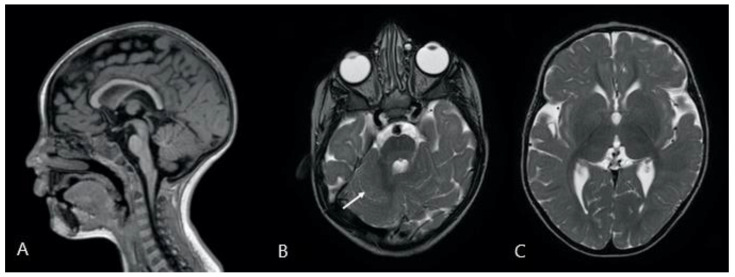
Description: (A): 3D-T1-weighted midline sagittal image shows a reduced thickness of the corpus callosum; the pons appears to be small and short compared to the length of the midbrain and medulla oblongata. The vermis is slightly hypoplastic with a prevalent reduction in height. (B,C): Turbo Spin Echo T2-weighted axial images showing (B) dysplastic cerebellum with abnormal foliation (white arrow), and (C) dysmorphic lateral ventricles, especially the frontal and occipital horns.
